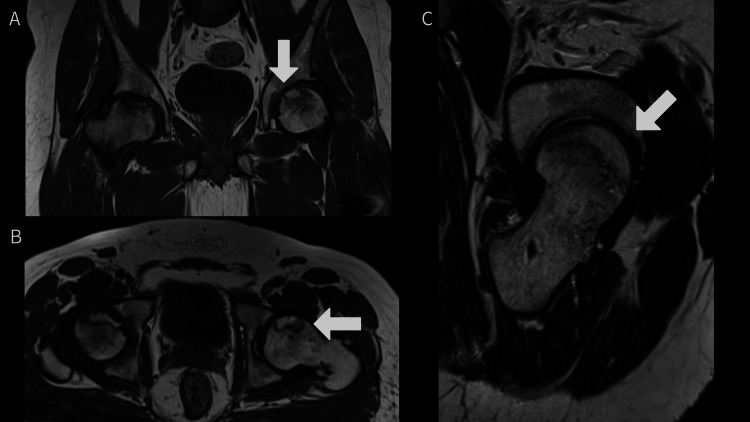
An MRI of the pelvis and bilateral hip joints was obtained, which showed features of Grade 2 AVN on the left side (marked with an arrow) and Grade 3 AVN on the right side MRI: magnetic resonance imaging; AVN: avascular necrosis
